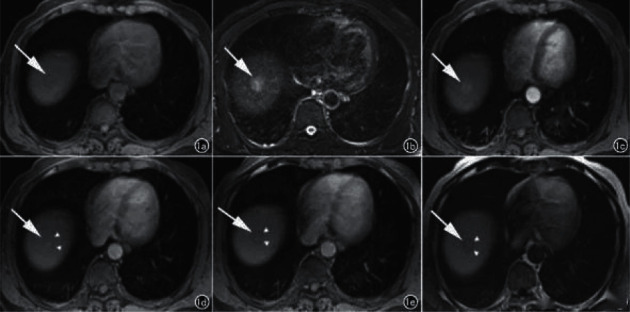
Axial MRI image (pulmonary enhancement) of a patient with HCC in the hepatic parietal region. (a) T1 low signal in the hepatic parietal region; (b) T2 slightly high signal nodule; (c) marked enhancement in the arterial phase; (d) portal venous phase; (e) transitional phase; (f) hepatobiliary phase contouring with a distinct low signal ring around the nodule (d–f triangle).
